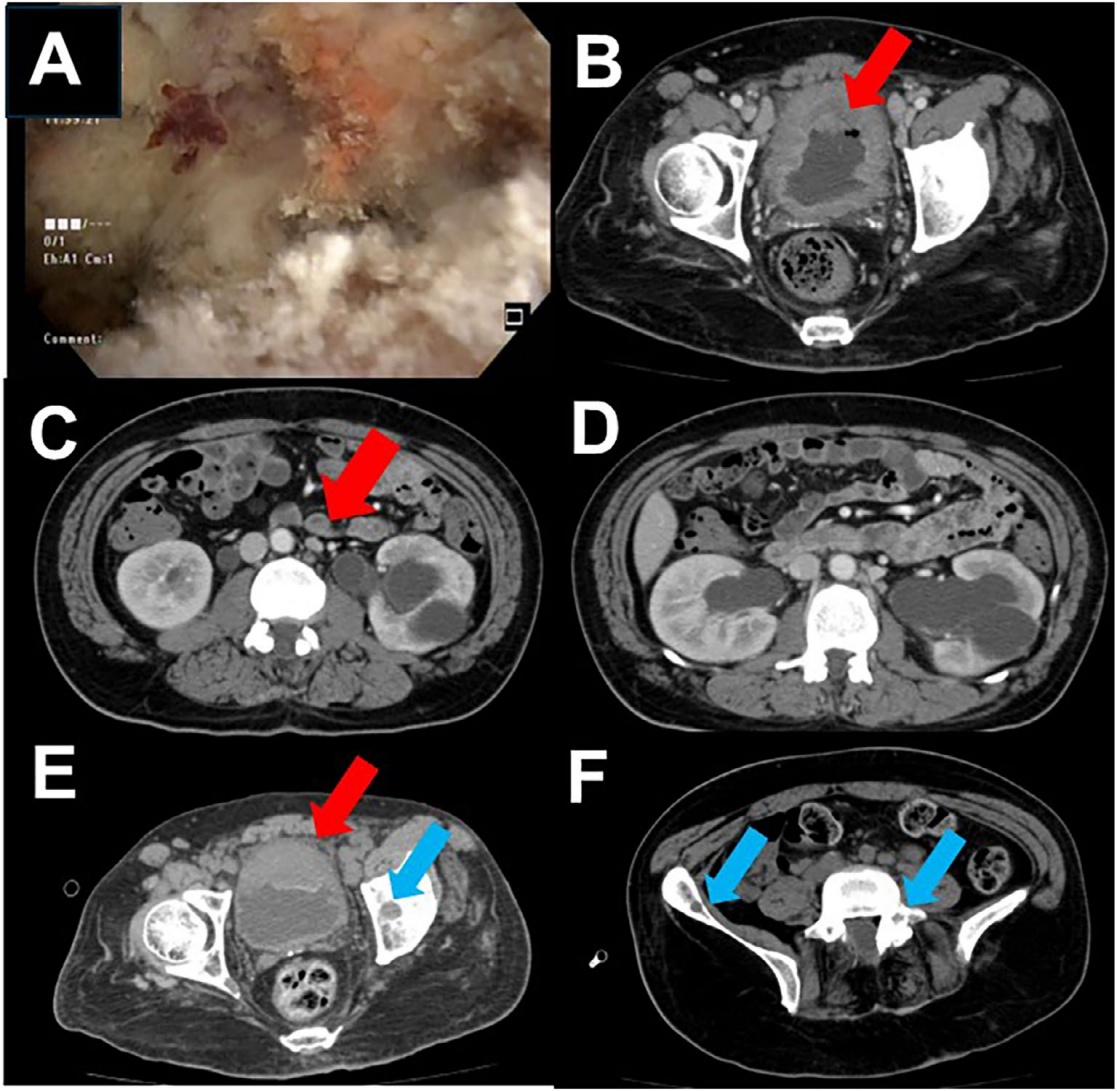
(A) Soft tissue covering the whole bladder including lesions suggesting papillary tumors. (B–D) Computed tomography revealed the cancerous lesion with infiltration of the extravesical bladder wall, multiple lymph node metastases, and bilateral hydronephroses. (E) The remaining bladder tumor (red arrow) and bone metastasis (blue arrow) are shown after two courses of gemcitabine and cisplatin. (F) Multiple pelvic bone metastases (blue arrows) appeared after chemotherapy.
